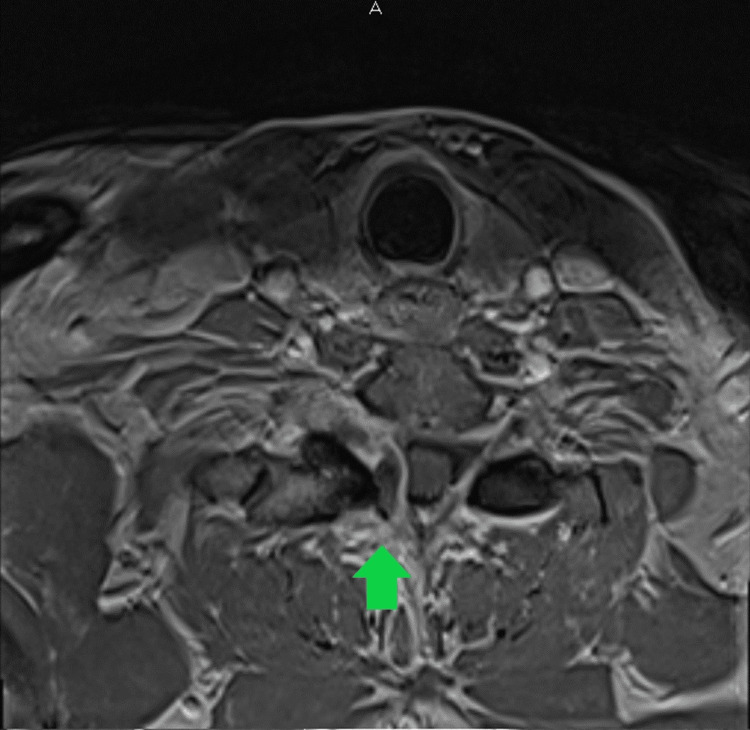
Contrast-enhanced axial MRI demonstrating the cyst.
